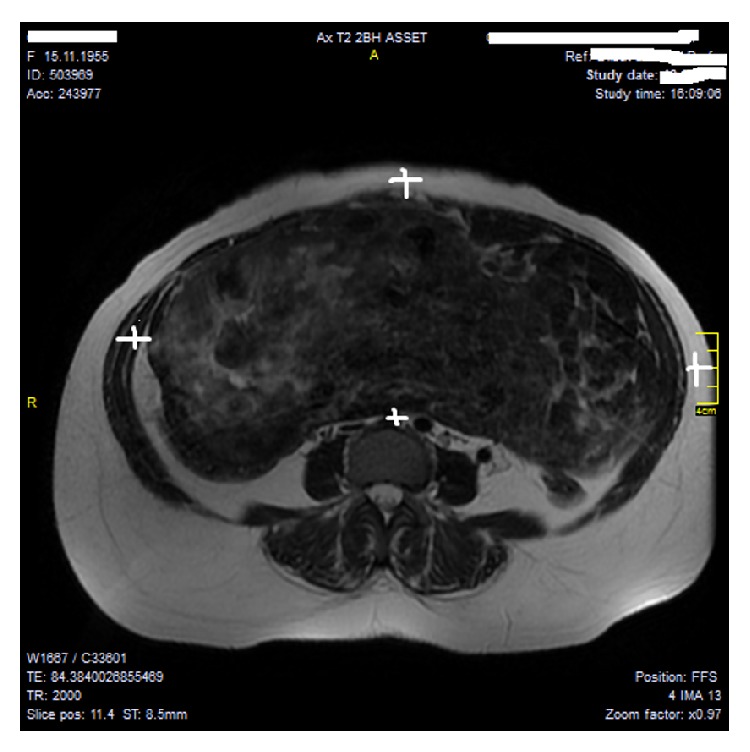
The figure shows MRI image of giant pelvic mass filling whole abdominal cavity with heterogenous and semisolid appearance (corresponding the image between four white callipers).
